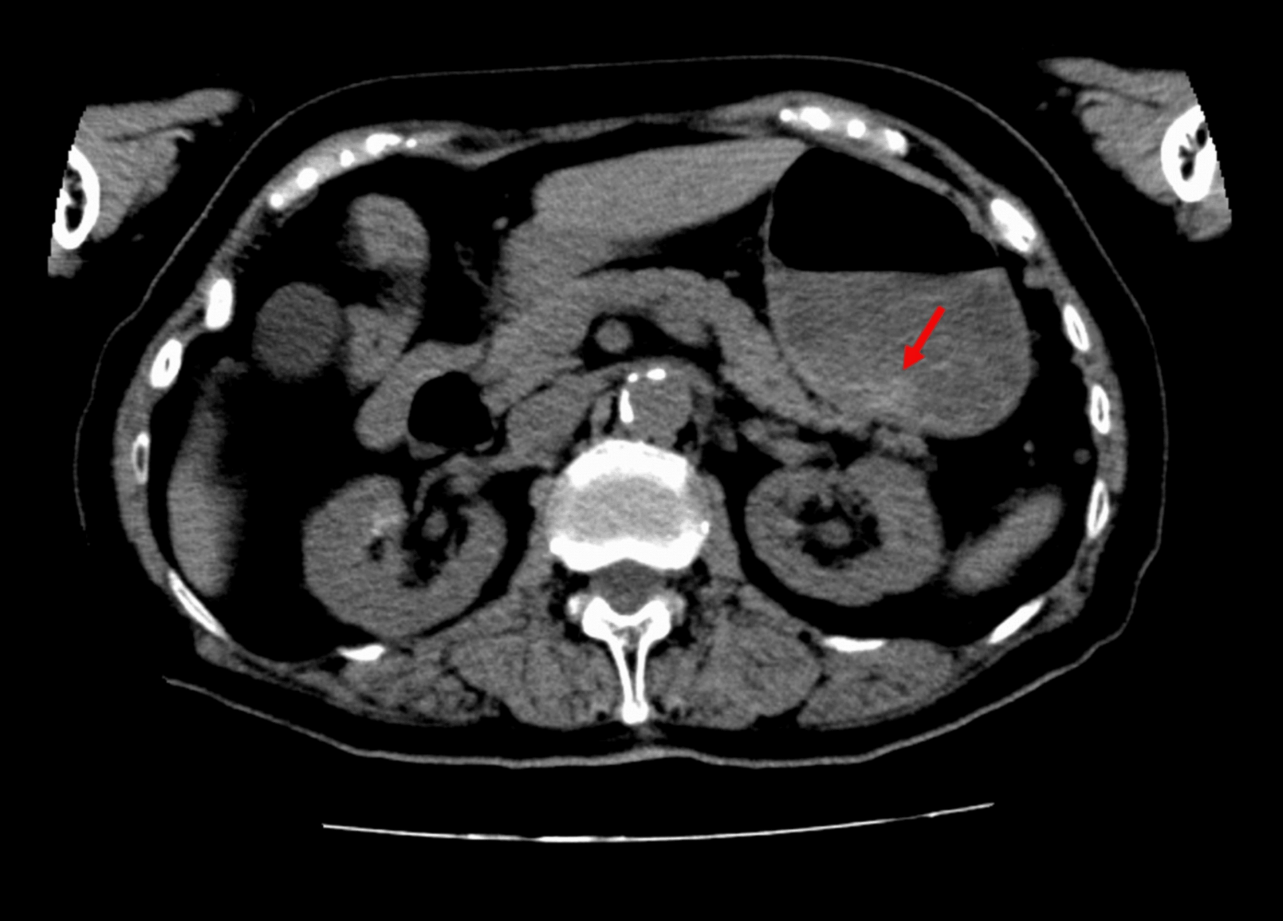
Abdominal CT image after admission The red arrow indicates an area of slightly elevated density in the stomach.
